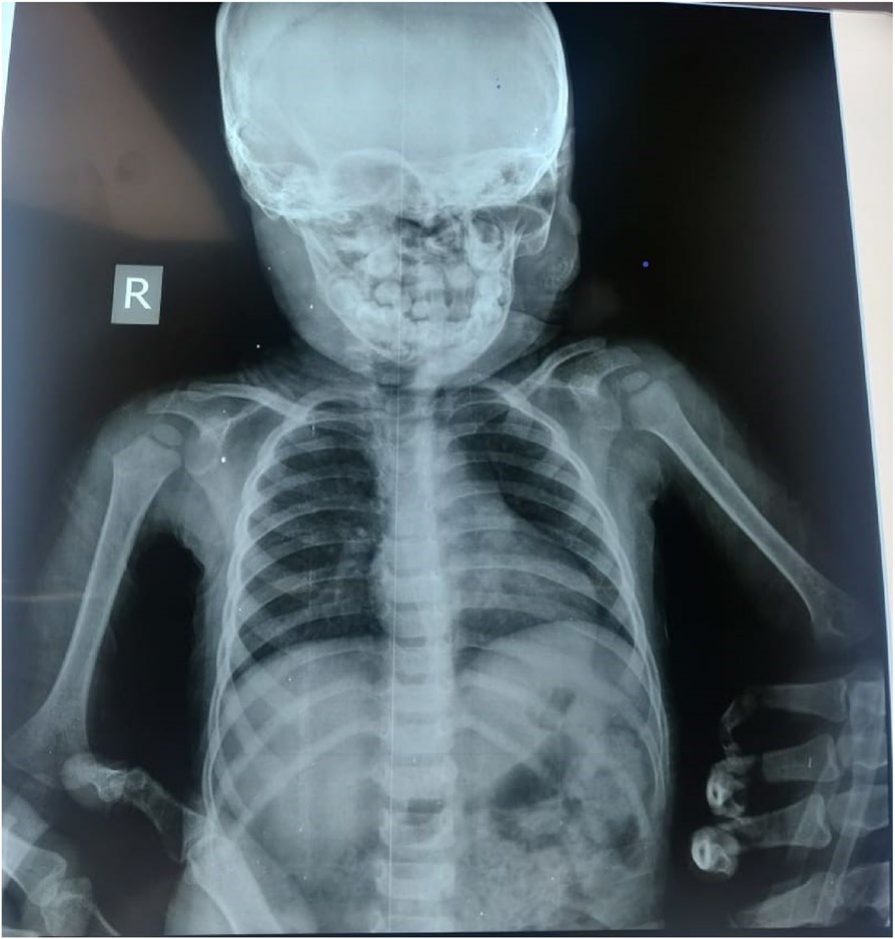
X-ray AP view showing position of toothbrush
